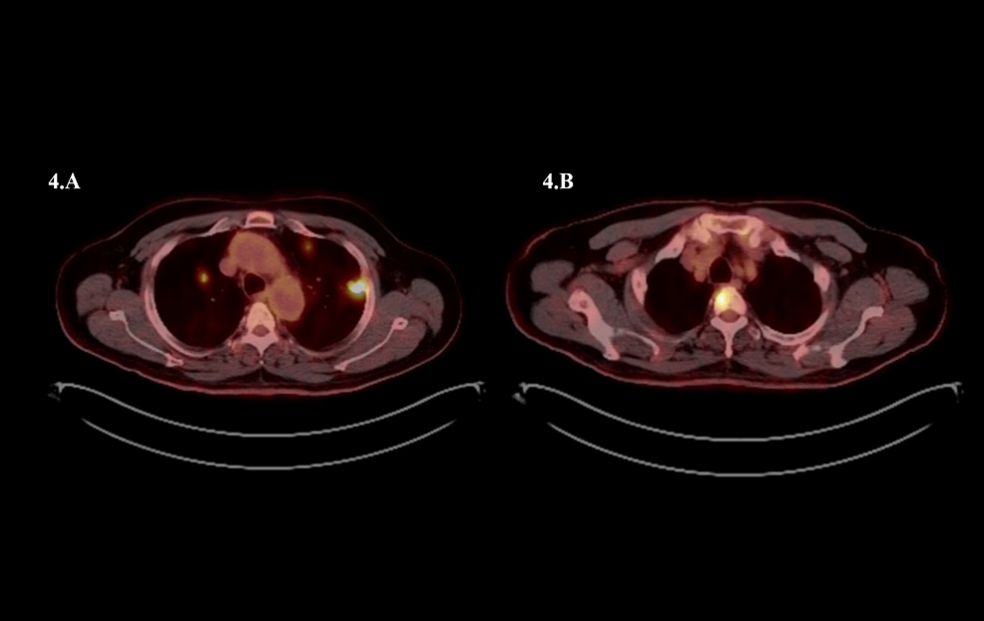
Positron emission tomography (PET) scan showing multiple F-fluorodeoxyglucose (FDG) avid pulmonary nodules (A) and bony lesions in the second thoracic vertebrae (B)
